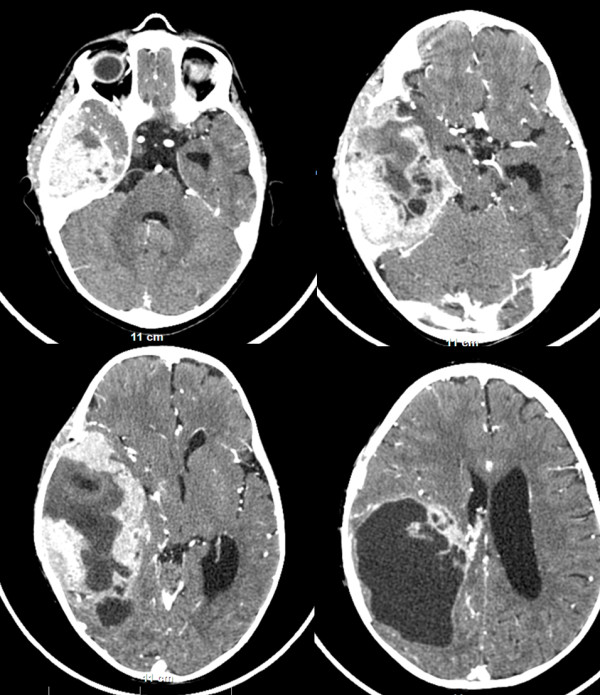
A contrast-enhanced computed tomography scan two months after the first operation, revealing a large (110×61mm) right temporoparietal, contrast-enhancing mass, with a partial cystic component and significant brain compression. The middle fossa and the parietal dura mater are invaded by the tumor. There is no significant brain edema.
